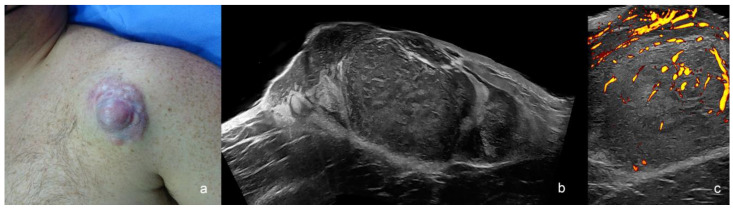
Dermatofibrosarcoma protuberans (a–c). (a) Clinical image. (b,c) Ultrasound images at 24 MHz ((b), greyscale panoramic view; (c). power Doppler; transverse views) present dermal and hypodermal mass with hypoechoic and hyperechoic areas that show pseudopods in the fatty tissue. On power Doppler, there is increased vascularity within the mass.
